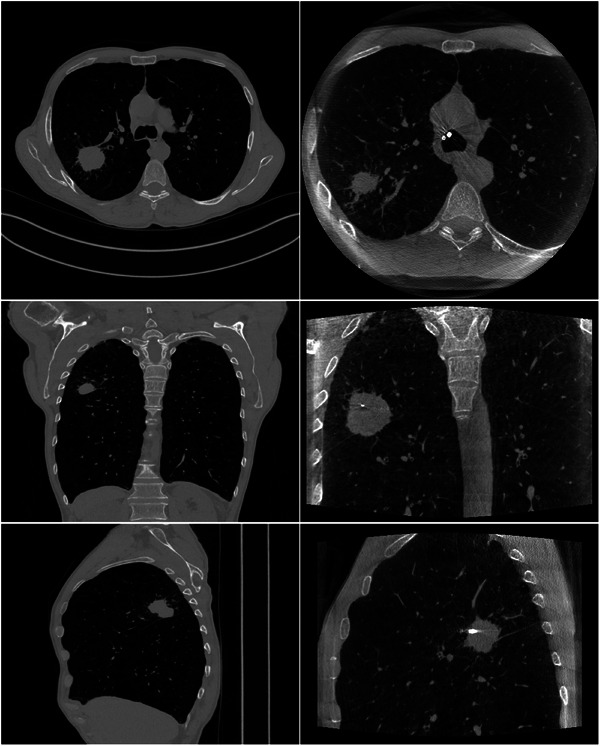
Patient bronchoscopy data. (a) Prior CT and (b) intraoperative CBCT. Upper row: one axial slice. Middle row: one coronal slice. Lower row: one sagittal slice. The display window range is [−1000 HU, 1000 HU]. CBCT, cone‐beam computed tomography; CT, computed tomography.
